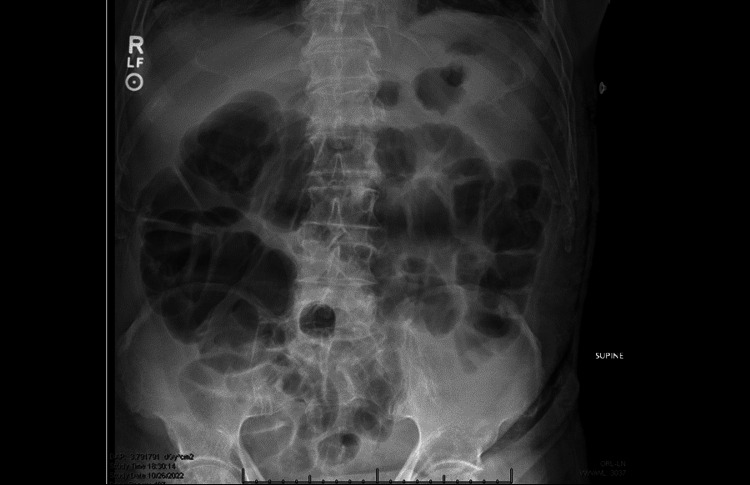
Abdominal X-ray from POD 1 showing ileus gas pattern with gaseous distention of several loops of the small and large bowel POD: postoperative day
